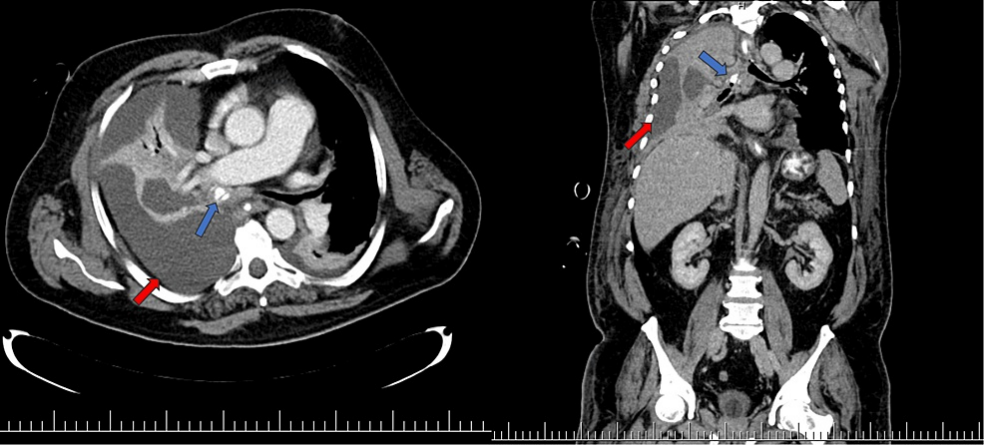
Axial and coronal CT image of the lung with intravenous contrast on 8 April 2019 revealing a calcifying lesion in right main bronchus (blue arrows) and pleural effusion (red arrows)
